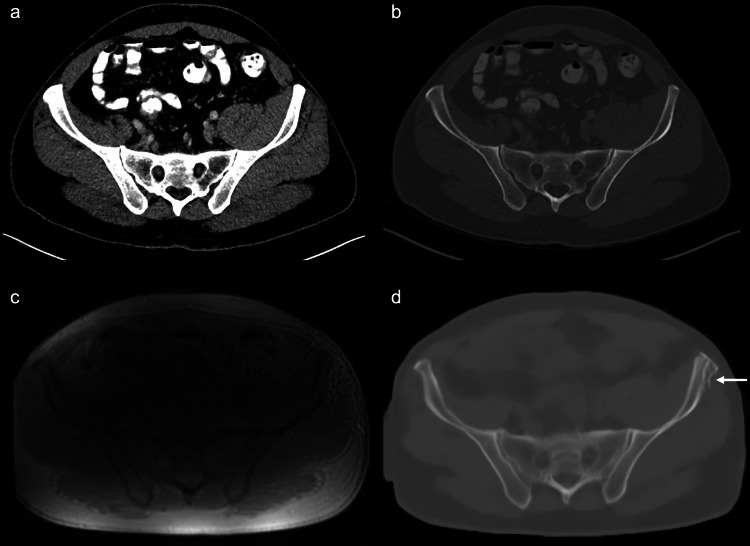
Exemplary CT (a, b), ZTE MRI (c), and pCT (d) images of the pelvis of a 45-year-old study participant. The pCT (d) image was generated from ZTE MRI (c) by applying a deep-learning algorithm. Note the almost perfect body masking and the small area that was falsely classified as “bone” (arrow) in (d)
